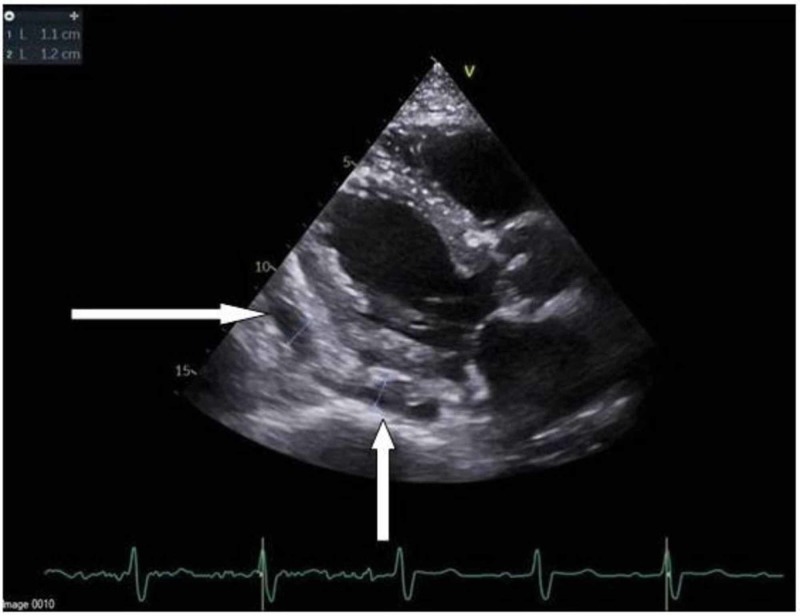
PLAX view of echocardiogram Thrombosed pseudoaneurysm demonstrated measurements in the top-left corner by arrows PLAX, parasternal long axis
